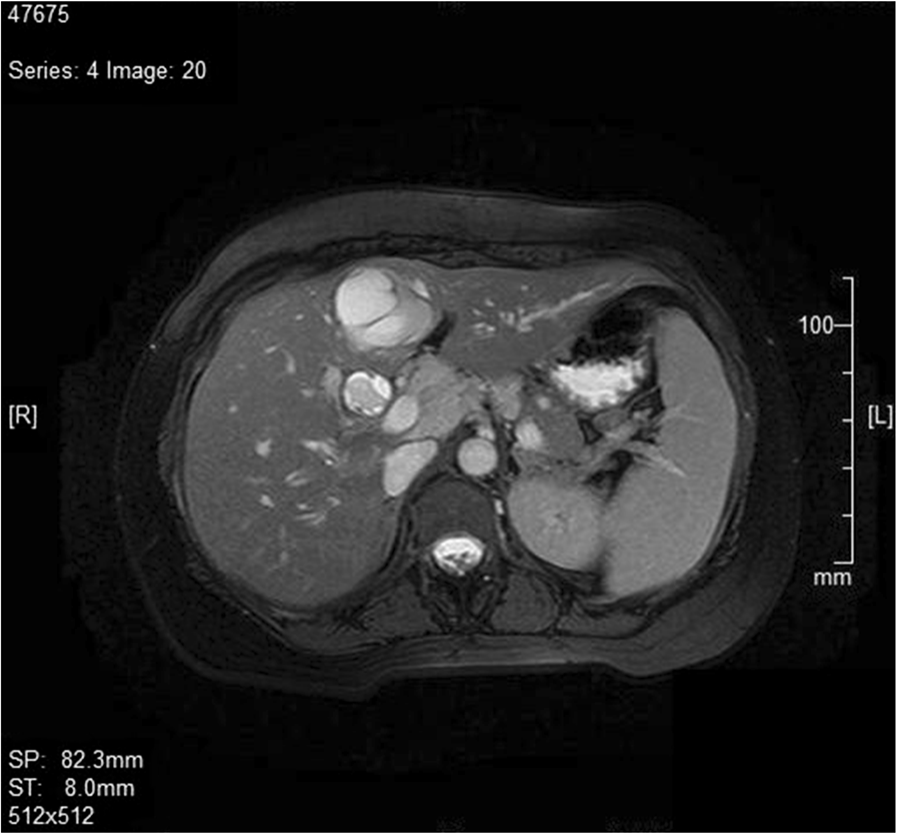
Magnetic resonance imaging image of the liver showing the dilated common bile duct with a filling defect within it indicating the tumor extending.
